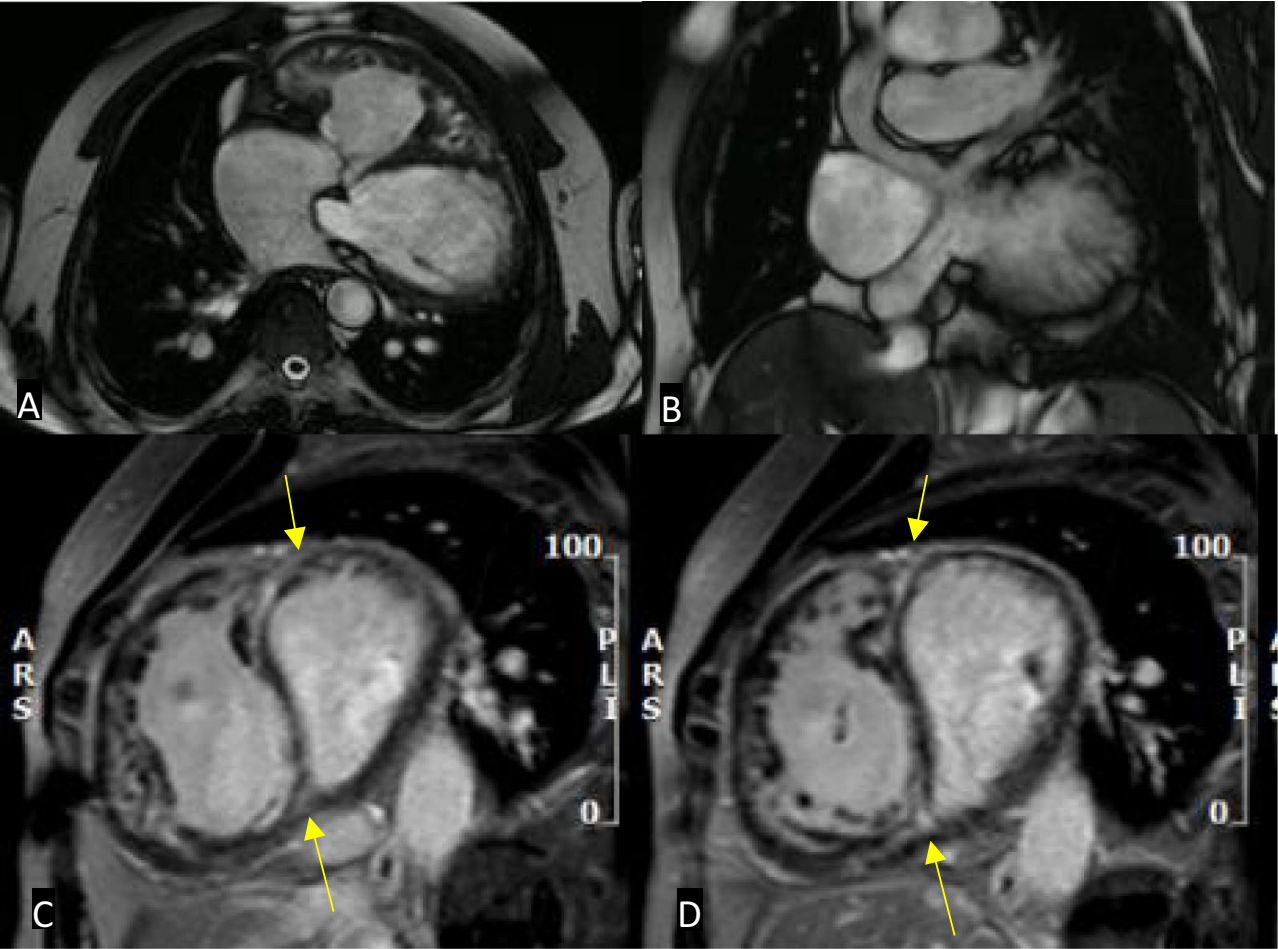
Still shots of MRI SSFP cine in axial (A), coronal (B) planes and mid-ventricular short-axis views of inversion recovery sequence in the late gadolinium phase (C, D) demonstrating the appearances of the heart post-atrial switch procedure for D-TGA. A Normal appearances of the baffled pulmonary venous system to the right atrium and B venae cavae to the left atrium. C, D Right ventricular dilatation and hypertrophy with late gadolinium enhancement at the insertion points extending into the mid-septum (yellow arrows)
